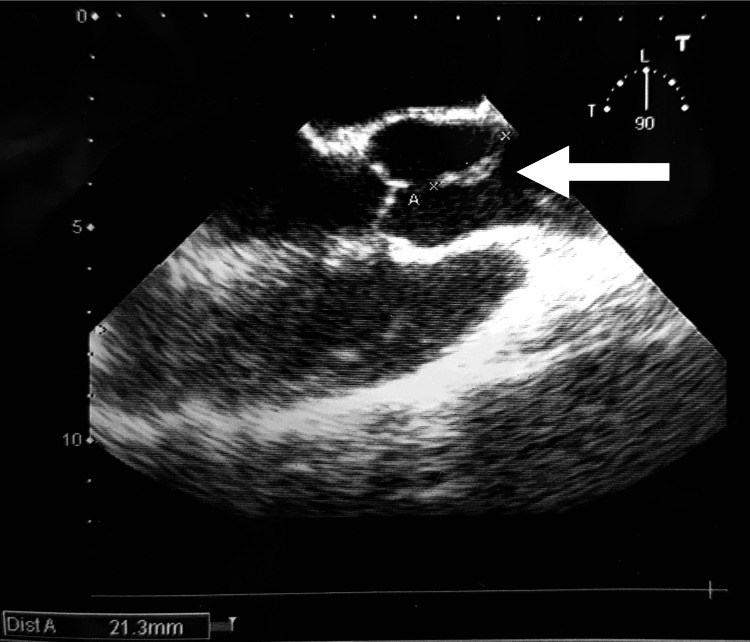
Transesophageal echocardiography shows a highly echogenic, flap-like structure in the ascending aorta (white arrow). Acute aortic dissection was suspected based on these findings.
